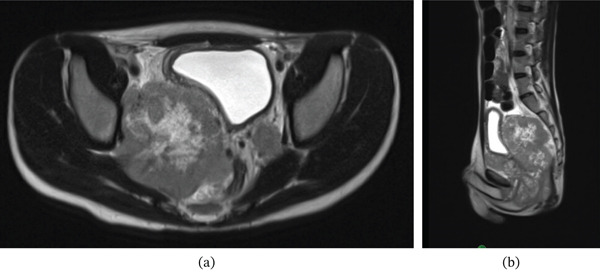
Select axial (a) and sagittal (b) MRI images demonstrating a large heterogeneous pelvic mass involving the peritoneum, bladder, and ilium.
